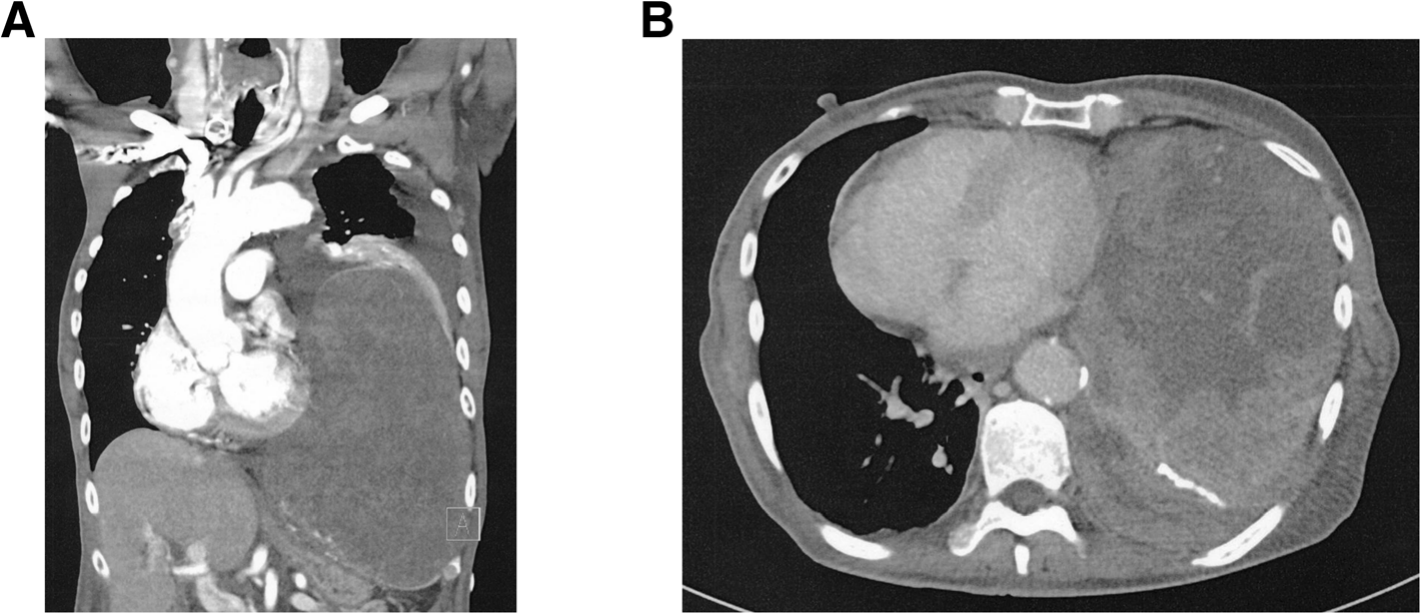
Preoperative computed tomography scan. a Coronal plane; a giant tumor occupied patient’s left pleural cavity and compressed the lung. b Axial plane
